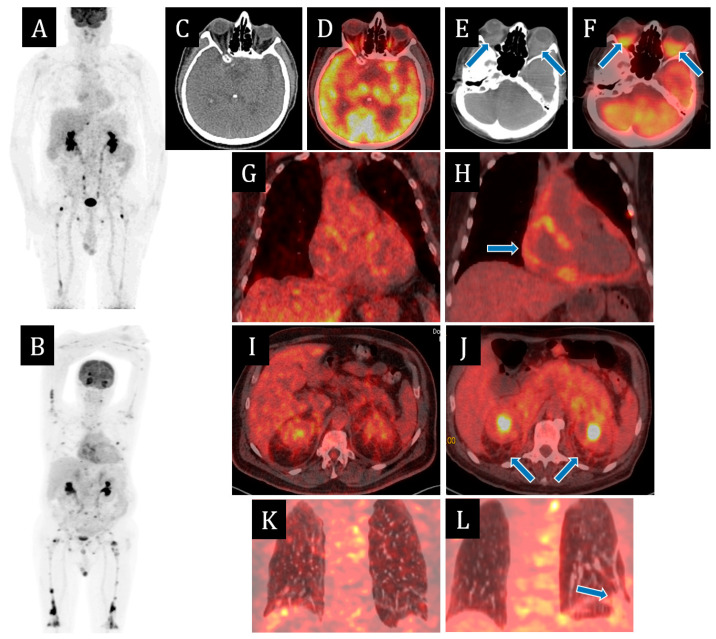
Positron emission tomography/computed tomography (PET/CT) images with [18F]-fluorodeoxyglucose ([18F]-FDG) on 9 March 2022 (A,C,D,G,I,K) and on 27 July 2023 (B,E,F,H,J,L). The maximum intensity projection (MIP) images (A,B) summarize the physiological and pathological distribution of ([18F]-FDG) in the body. PET/CT scans on 27 July 2023 showed the negative dynamics in the form of metabolically active changes in retrobulbar fibres (E,F), appearance of metabolic active pericardial effusion (H) and consolidation zones in S9, S10 of the left lung (L), the increase in the number and metabolic activity of foci in skeletal bones (B) and the increase of pronounced changes in retroperitoneal fibre (J).
